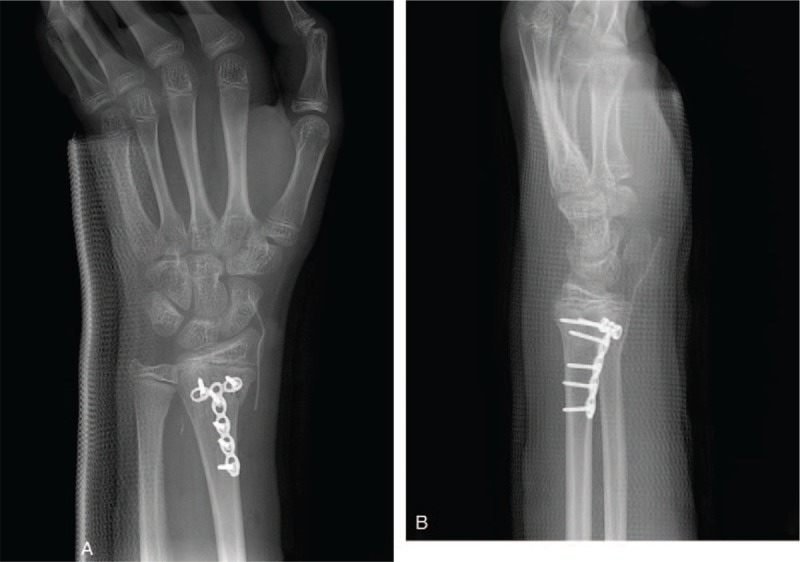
Postoperative posteroanterior and lateral plain radiographs showing volar plate fixation of the Smith fracture.
